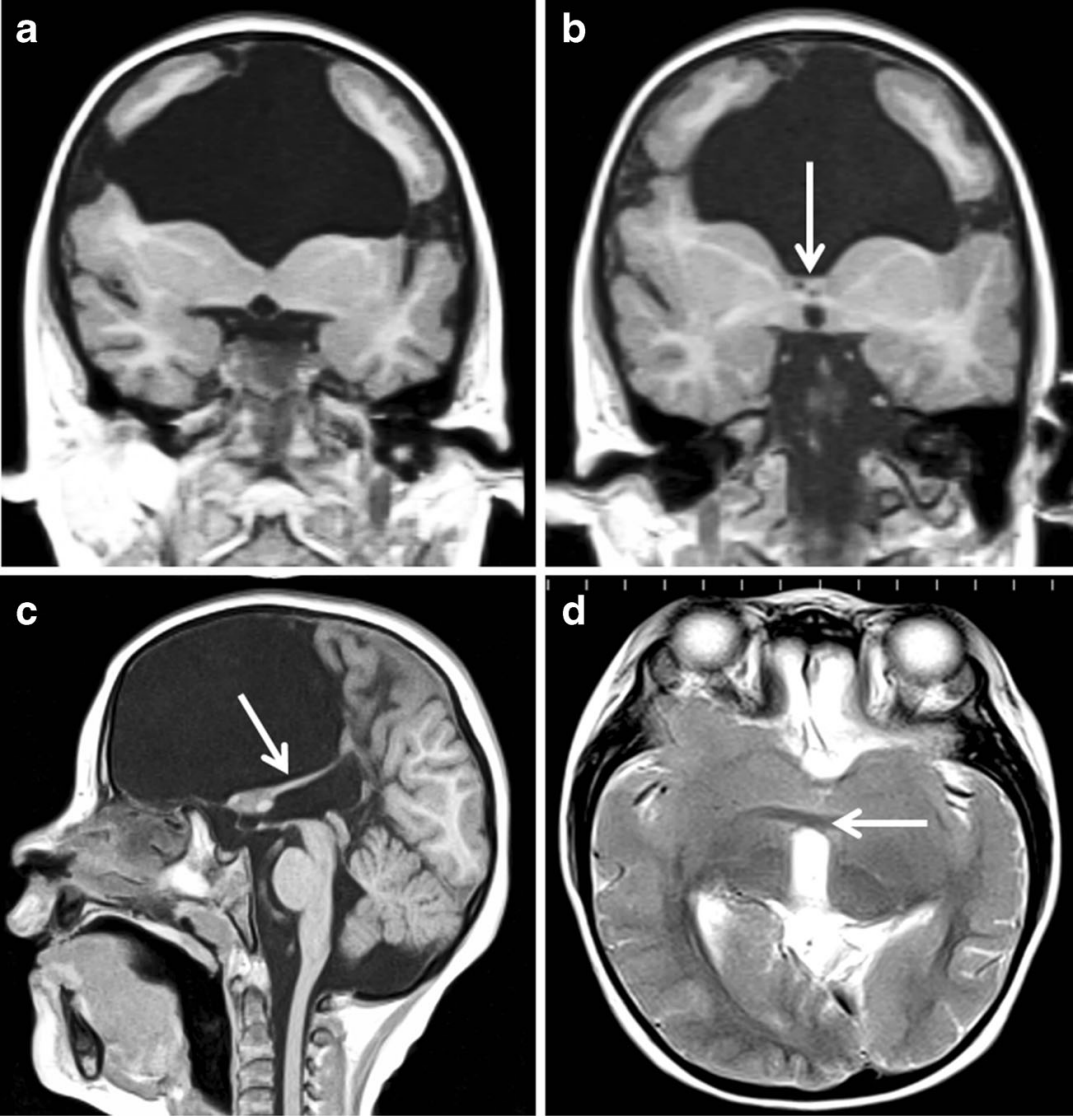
Bilateral schizencephaly (type 3) with multiple other brain abnormalities. MR imaging of a 5-year-old child with global developmental delay and epilepsy. Coronal reconstructions from T1 volume datasets (a, b) show bilateral schizencephaly (type 3) involving the inferior frontal gyri. Bilateral cortical formation abnormalities are present in the superior portions of the frontal lobes. The corpus callosum and septum pellucidum are absent and the fornices have an abnormal low position (arrowed on b and c). Axial T2-weighted image shows abnormal fusion of the nucleus accumbens septi and basal forebrain across the midline (arrowed on d) which possibly represents a form of septo-preoptic holoprosencephaly
